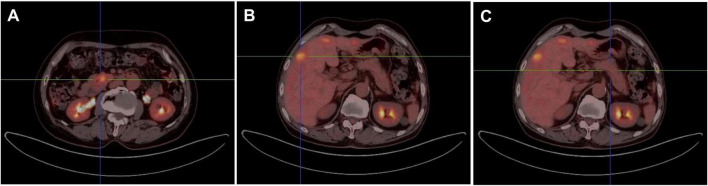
Imaging of the duodenum (A), liver (B) and pancreas (C) with PET/CT.
